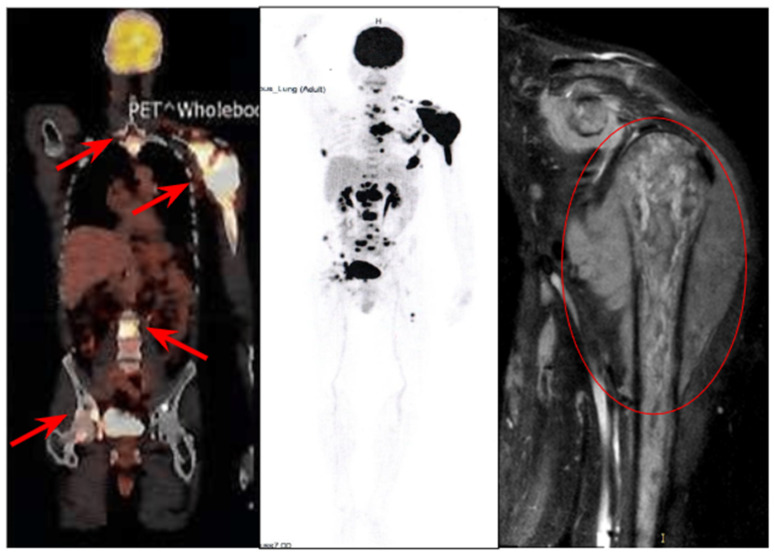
Patient’s baseline 18F-fluorodeoxyglucose (FDG)—positron emission tomography (PET) with computed tomography (CT), February 2020. Computed tomography (CT) provides detailed visualization of the soft tissue mass surrounding the left shoulder, which is outlined with a red line. PET images demonstrate multiple lesions, including involvement of the articular process of the left scapula, a mass in the 5th intercostal space with vertebral bone destruction and extension into the spinal canal, multiple cervical lymph node lesions, and widespread bone metastases.
